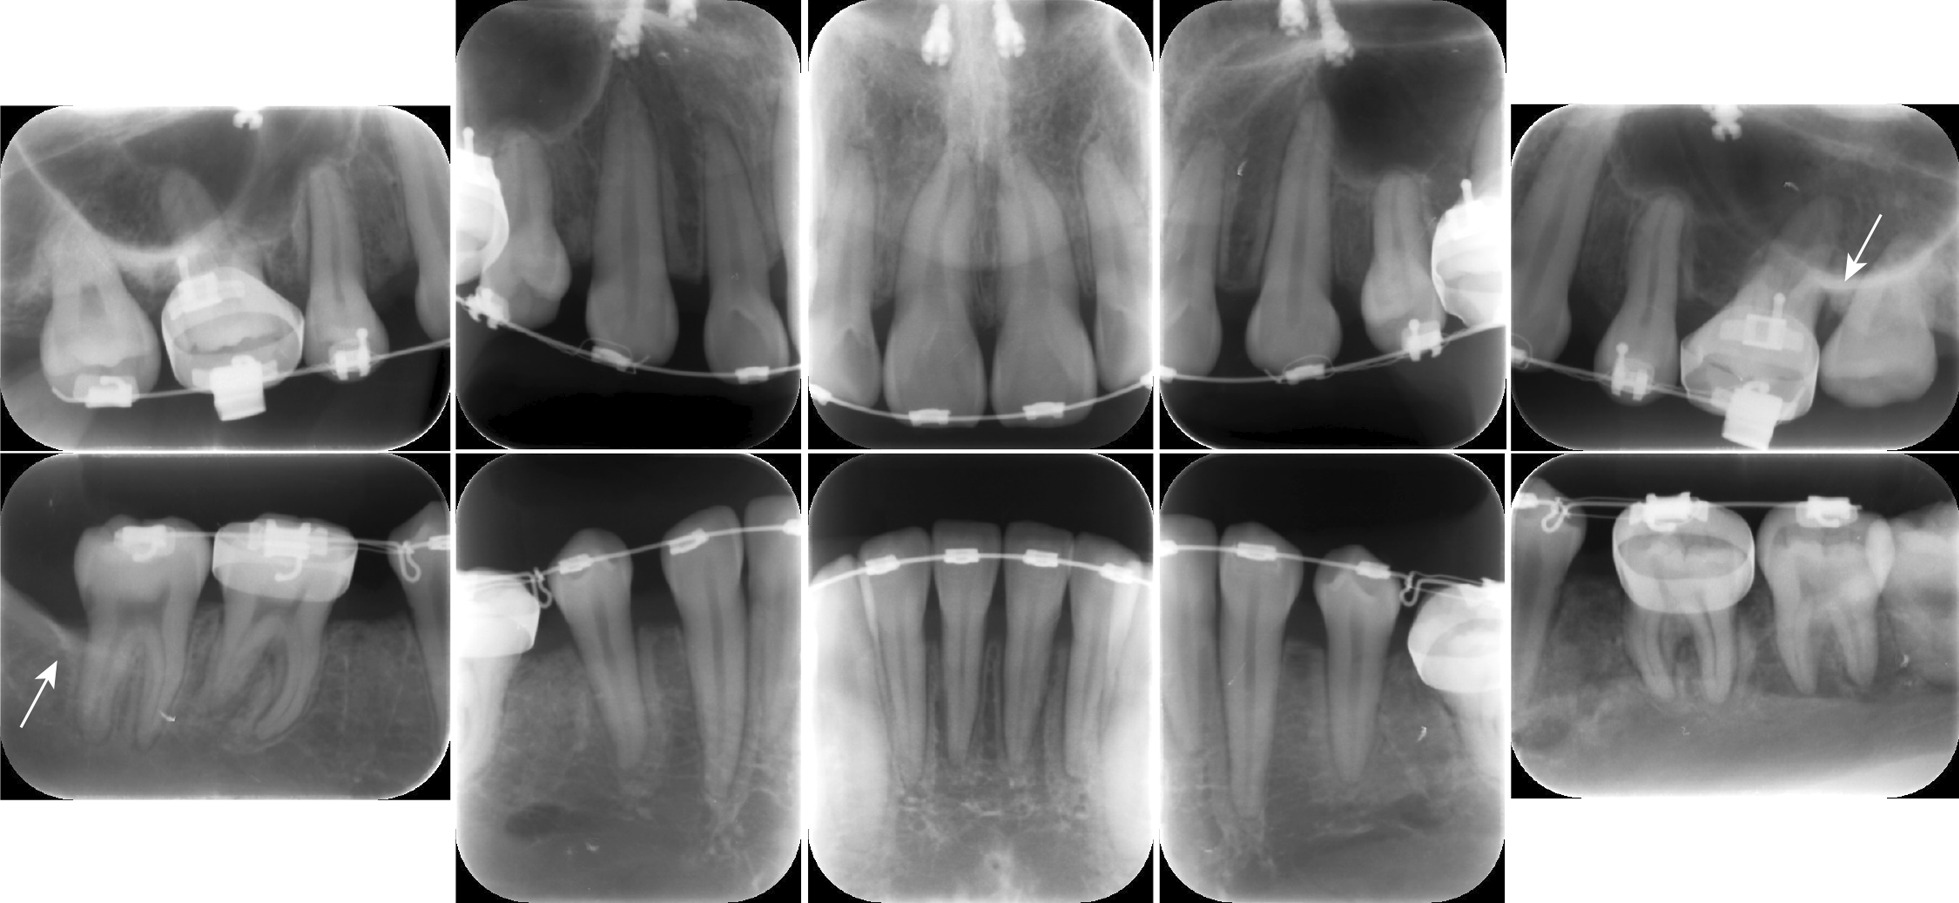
Intraoral periapical radiographs. White arrows indicate vertical bone loss
